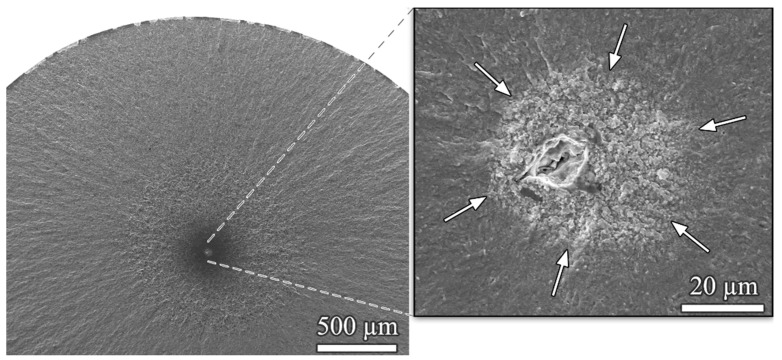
Fatigue crack initiation in 100Cr6 bearing steel at an interior non-metallic inclusion after ultrasonic fatigue testing at *σ*_a_ = 875 MPa, *R* = −1, *N*_f_ = 2.4 × 10^9^ cycles; the border of FGA is marked with arrows.
